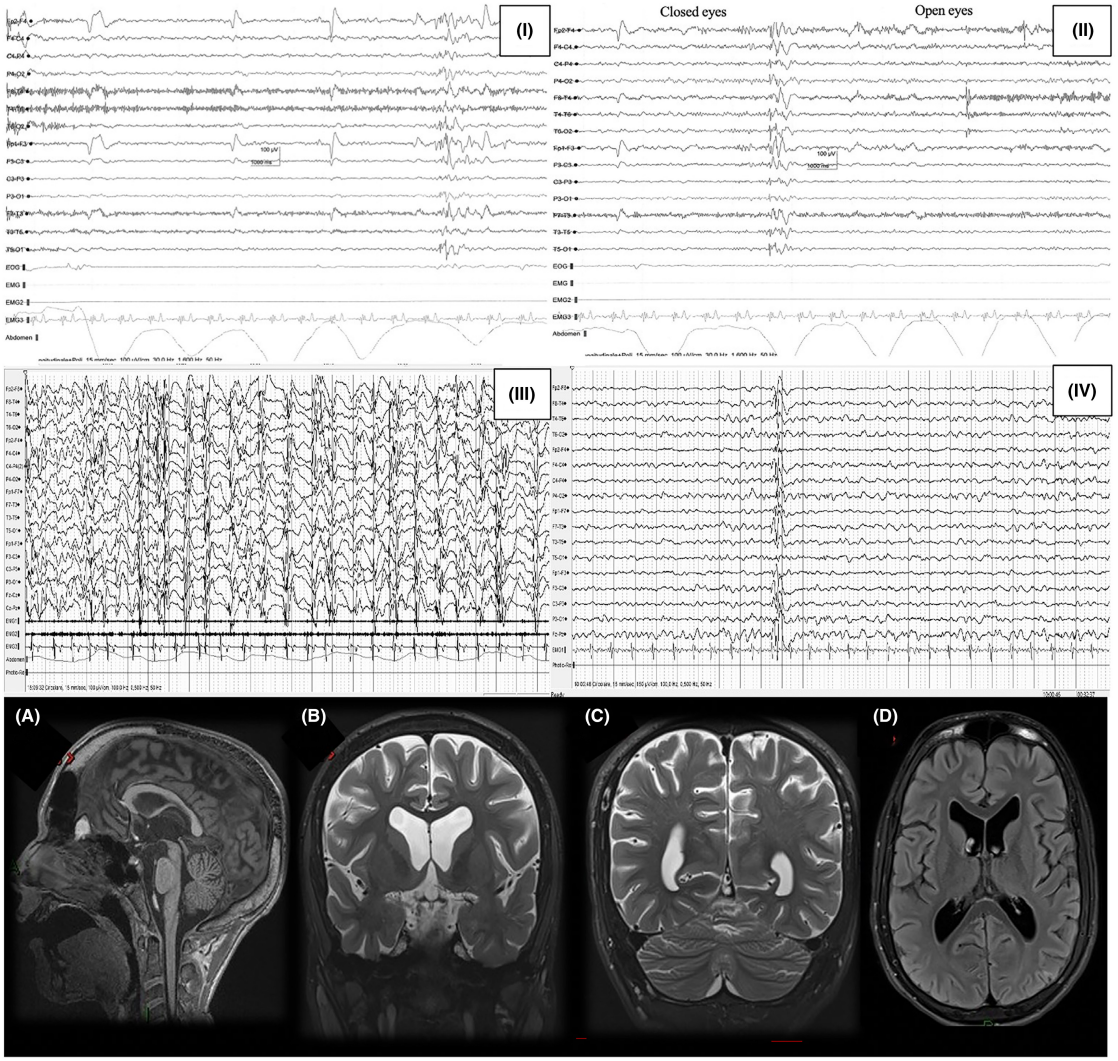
Electroencephalogram (EEG) and magnetic resonance imaging (MRI) of Pts. 3 and 4. Panels I and II show the EEG of Pt. 3 and Pt. 4, respectively (both at 16 years): they display a common pattern of poor general organization, prevalence of diffuse theta activity, poor reactivity to eye closure, and rare spikes or spikes‐and‐waves with frontal predominance; poor sleep organization without recognizable sleep phases was also observed. Panel III (Pt. 3, 12 years) shows an EEG during sleep with sequences of spike‐and‐wave activation in sleep evolving as the patient grew. Panel IV shows a sleep EEG of Pt.3 at 17 years in a dedifferentiation between awake and asleep states, with no recognizable sleep figures. Pt. 3 Brain MRI (16 years). (A) T1 sequence, sagittal plane: we can observe a thin corpus callosum and brainstem. Cerebellar atrophy is also noticeable. (B) T2 sequence, coronal plane: cerebral atrophy with enlarged ventricles. (C and D) T2 sequence, coronal and horizontal plane: marked cerebral and cerebellar atrophy are evident.
